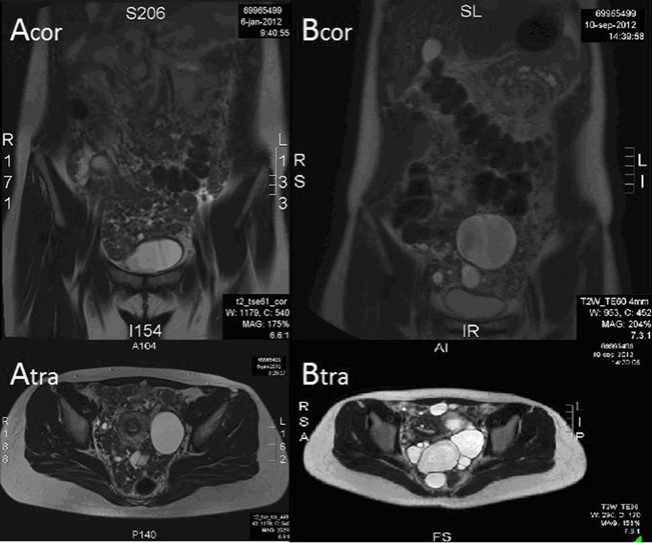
MRI view of LPD. *Acor* coronal view before treatment, *Atra* transverse view before treatment, *Bcor* coronal view after 3 months of treatment, *Btra* transverse view after 3 months of treatment
